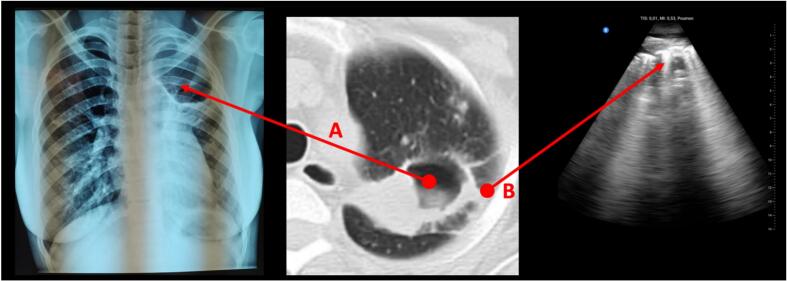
Arrow A; cavities are easily detected on CT-scan and chest X-ray. Arrow B; Gas-filled cavities remain invisible on lung ultrasound because of the reverberation artefact. However, their inflammatory boarders can be detected and can cause an interstitial or alveolar syndrome. Here we note multiple B-lines, a broken and irregular pleural line with subpleural consolidations.
